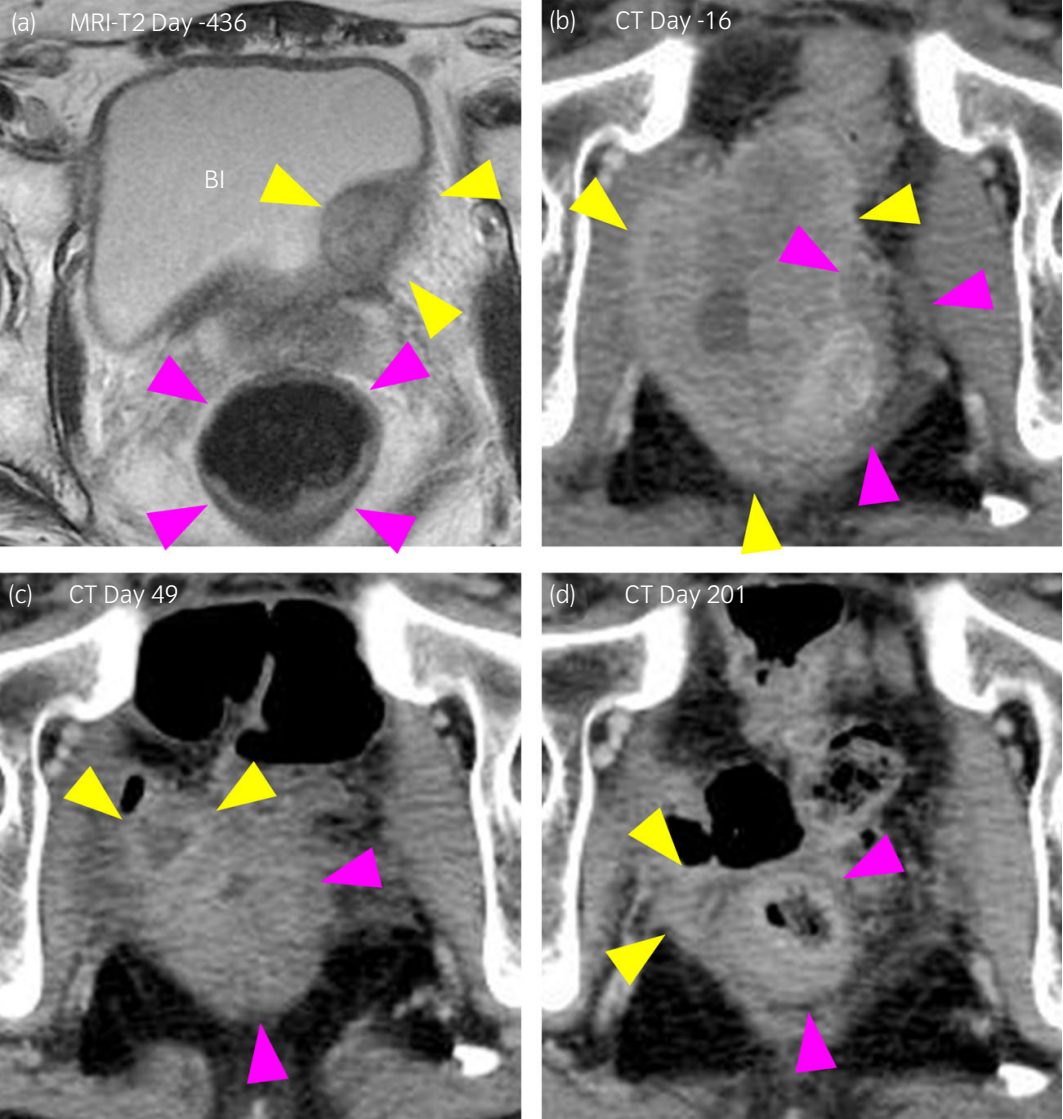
Imaging study of the case. (a) A T2‐weighted image of MRI on day 436. (b) CT performed before pembrolizumab treatment on day 16. (c, d) CT after pembrolizumab treatment on day 49 and 201, respectively. Yellow and pink arrowheads in panels (a–d) indicate bladder tumor (clinical T2–T3) and the rectum, respectively.
